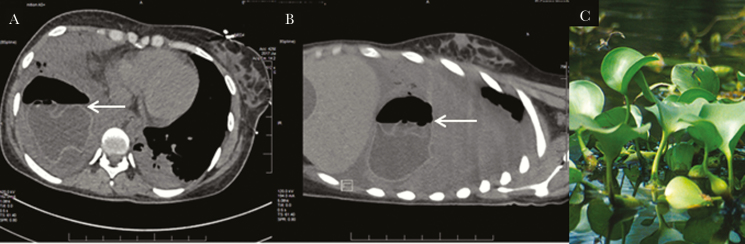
A, Computed tomography of chest, axial view, demonstrating ruptured hydatid cyst with air-fluid level and serpiginous parasitic membrane (arrow). B, Sagital view demonstrating crumpled parasitic membrane floating at the air-fluid level (arrow). C, Camalote plant (common water hyancinth), *Eichhornia crassipes*. Source: United States Department of Agriculture (www.ars.usda.gov/oc/images/photos/mar00/k8801-2, accessed August 10, 2018).
